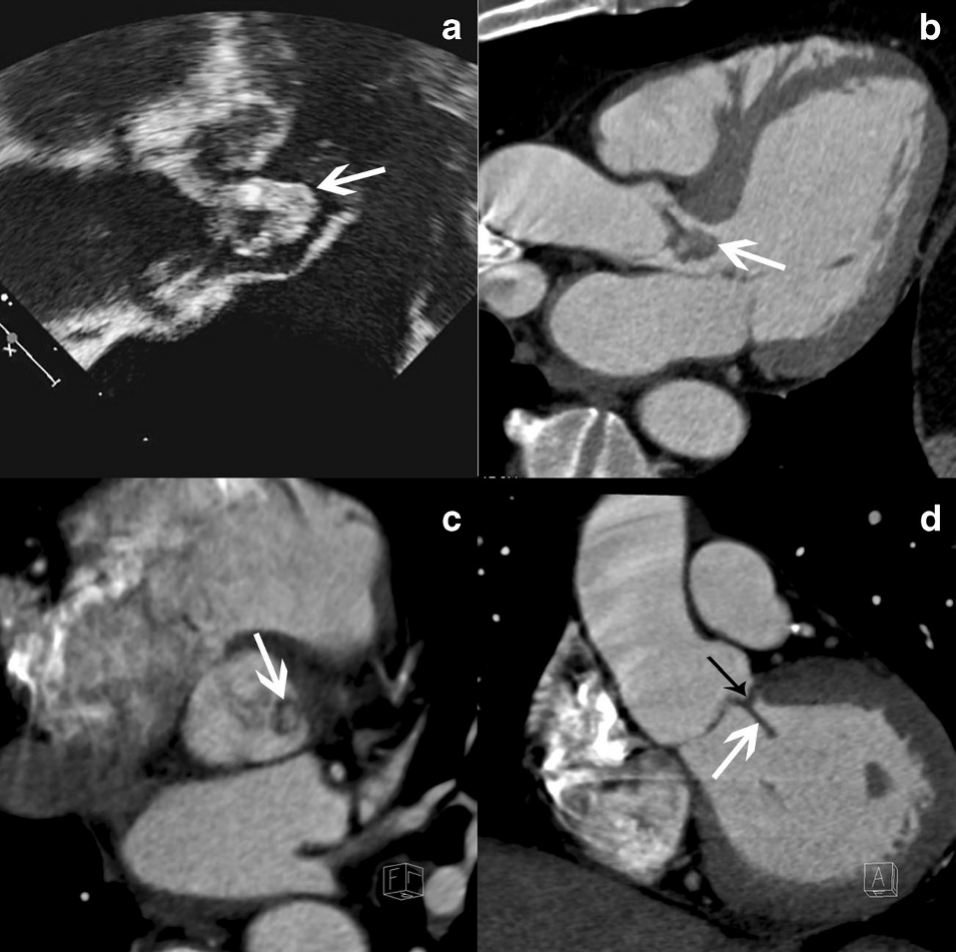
Results of echocardiography and MSCT studies in case of aortic valve infective endocarditis. Images show TEE studies, 120-degree long-axis view, at the level of the left ventricular outflow track (a) and MSCT acquisition with MPR reconstructions in LVOT view (b), aortic valve plane view (c) and coronal oblique view at the level of the aortic root (d) show a large vegetation (*white arrow*) and a valvular thickening of the right coronary aortic cusp (*black arrow*)
